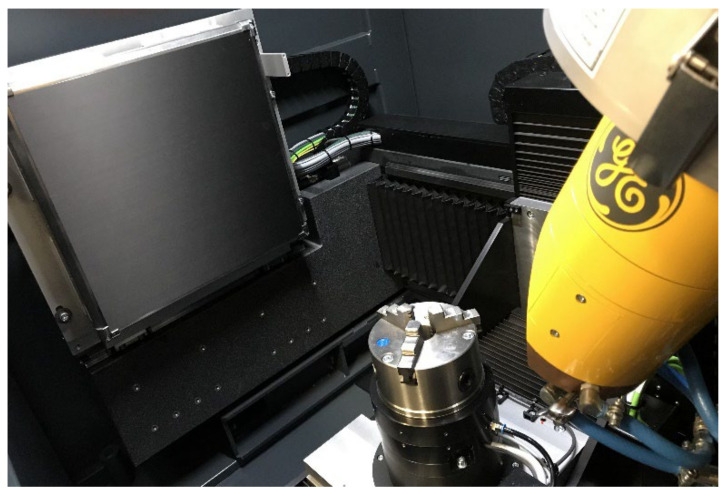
The tomographic chamber of the cone beam device.
